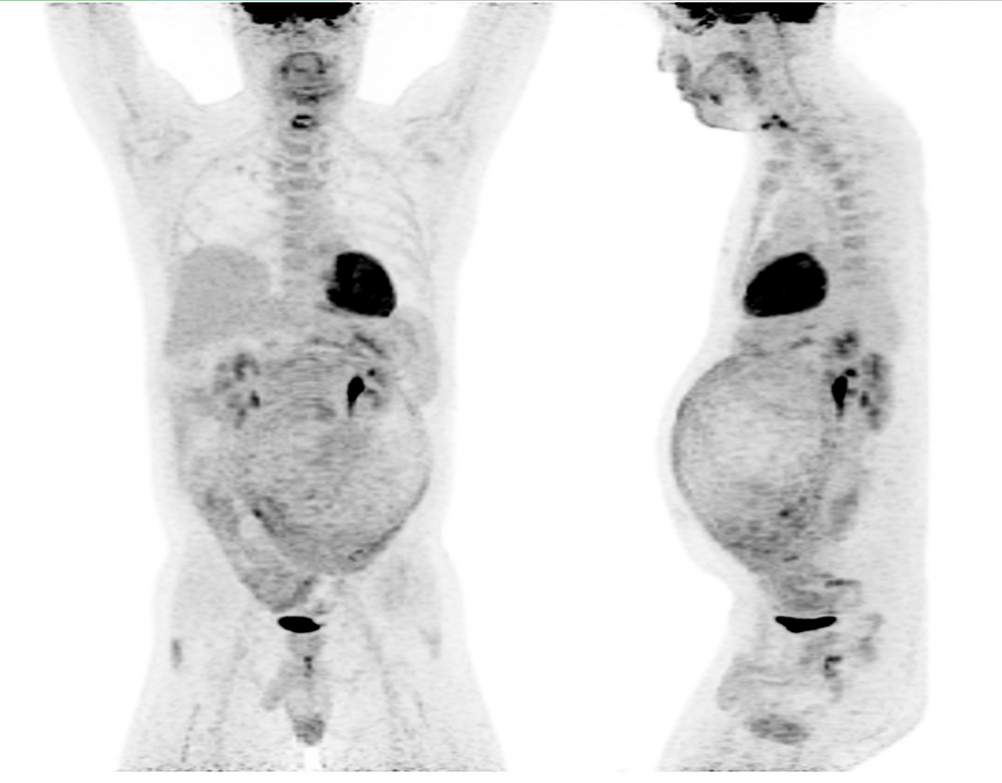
Post-radiation therapy positron emission tomography/CT scan (maximum intensity projection images) performed 4 weeks after the end of therapy showing excellent partial treatment response.
